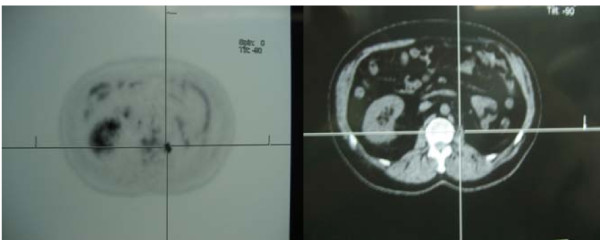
PET - CT demonstrating an area of increased uptake in the left paravertebral area.
